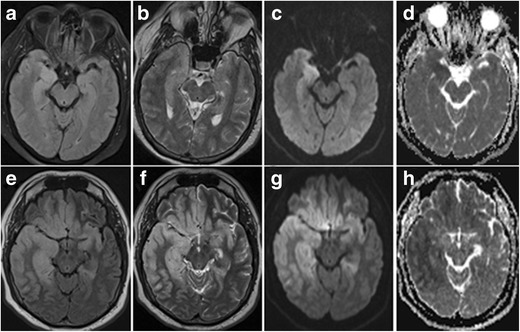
Early stage (a–d) versus more advanced (e–h) herpes encephalitis. 44-year-old male patient with fever, headache and acute epileptic seizures. 1.5-T axial T2W (a), FLAIR (b) and diffusion-weighted (c) images with an ADC map (d) show subtle T2-FLAIR hyperintensity in the right amygdala and hippocampus with diffusion restriction. Follow-up MRI performed one week later (e–h) shows more pronounced T2-hyperintense bilateral frontotemporal cortical oedema and diffusion restriction, more pronounced in the right hemisphere. PCR was positive for HSV
